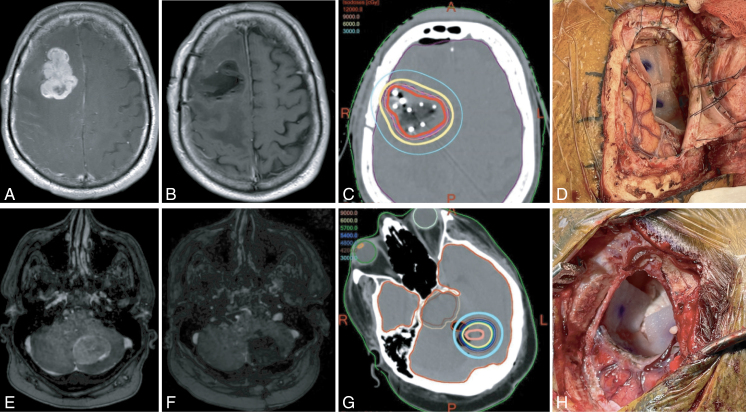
Case examples of 2 patients who underwent surgical resection and GammaTile placement for newly diagnosed brain metastases. A right frontal melanoma metastasis is demonstrated by the preoperative axial T1-weighted post-contrast MRI (A), immediate postoperative axial T1-weighted post-contrast MRI (B), GammaTile radiation therapy dosimetry (C), and intraoperative photograph of resection cavity lined with GammaTile (D). A left cerebellar non–small cell lung cancer metastasis is demonstrated by the same images, respectively (E, F, G, H).
